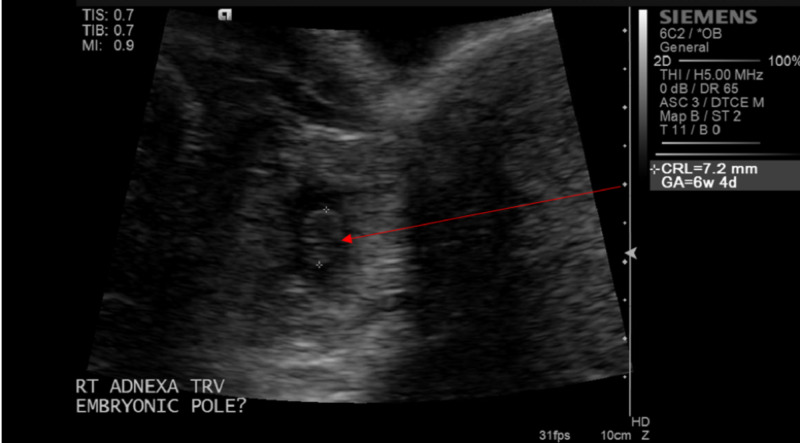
Pelvic ultrasound (transverse view): ectopic pregnancy with a crown-rump length (CRL) of 7.2 mm (gestational age six weeks and four days) in the right adnexal area
